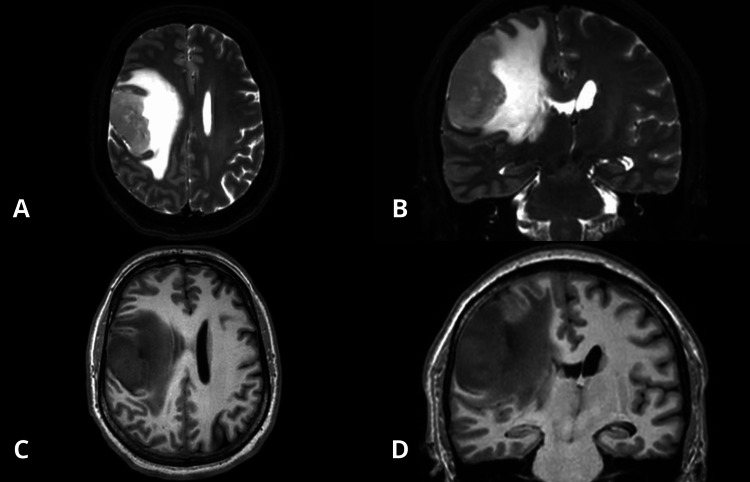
Contrast-enhanced T2 and T1 MRI scan of the skull A: contrast-enhanced T2 axial MRI scan of the skull showing a right frontoparietal lesion with a neoplastic appearance; B: contrast-enhanced T2 coronal MRI scan of the skull showing a right frontoparietal lesion with a neoplastic appearance; C: contrast-enhanced T1 axial MRI scan of the skull showing a right frontoparietal lesion with a neoplastic appearance; D: contrast-enhanced T1 coronal MRI scan of the skull showing a right frontoparietal lesion with a neoplastic appearance.
